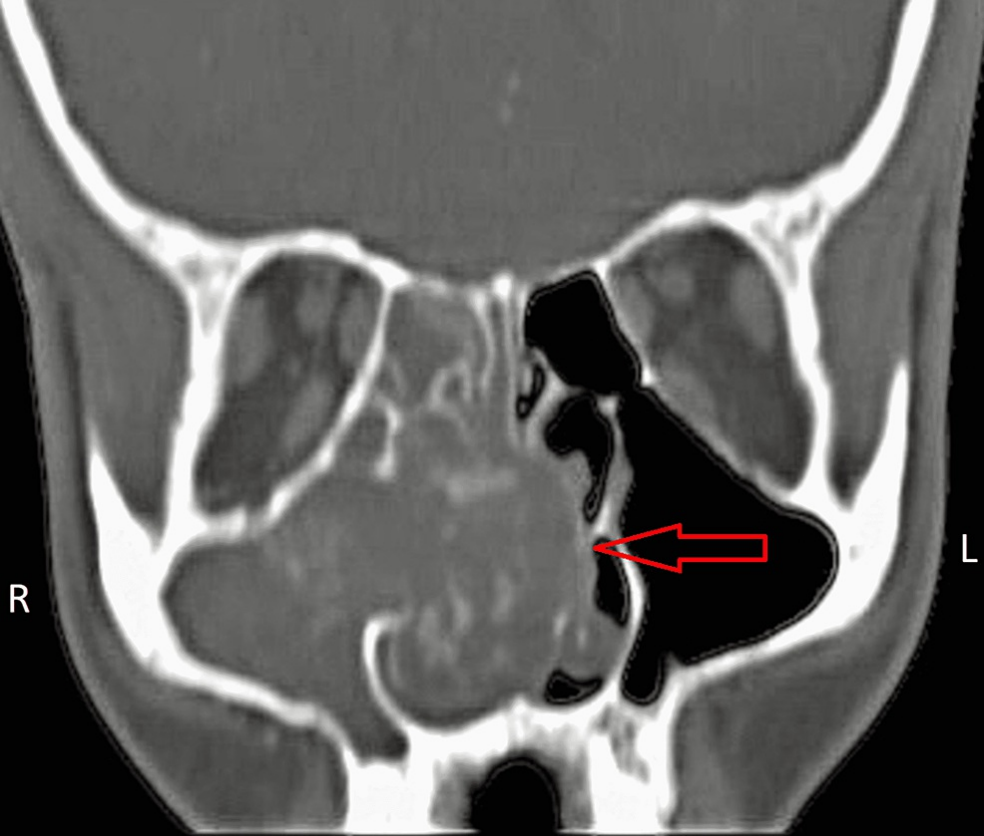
Coronal plane CT-PNS image showing, soft tissue density lesion completely occluding right maxillary sinus, entering the right nasal cavity through the widened ostium with mass effect on the nasal septum resulting in deviated nasal septum with convexity toward the left side causing partial obstruction of the left nasal cavity and atrophy of the corresponding middle turbinate due to compression. PNS - paranasal sinuses
